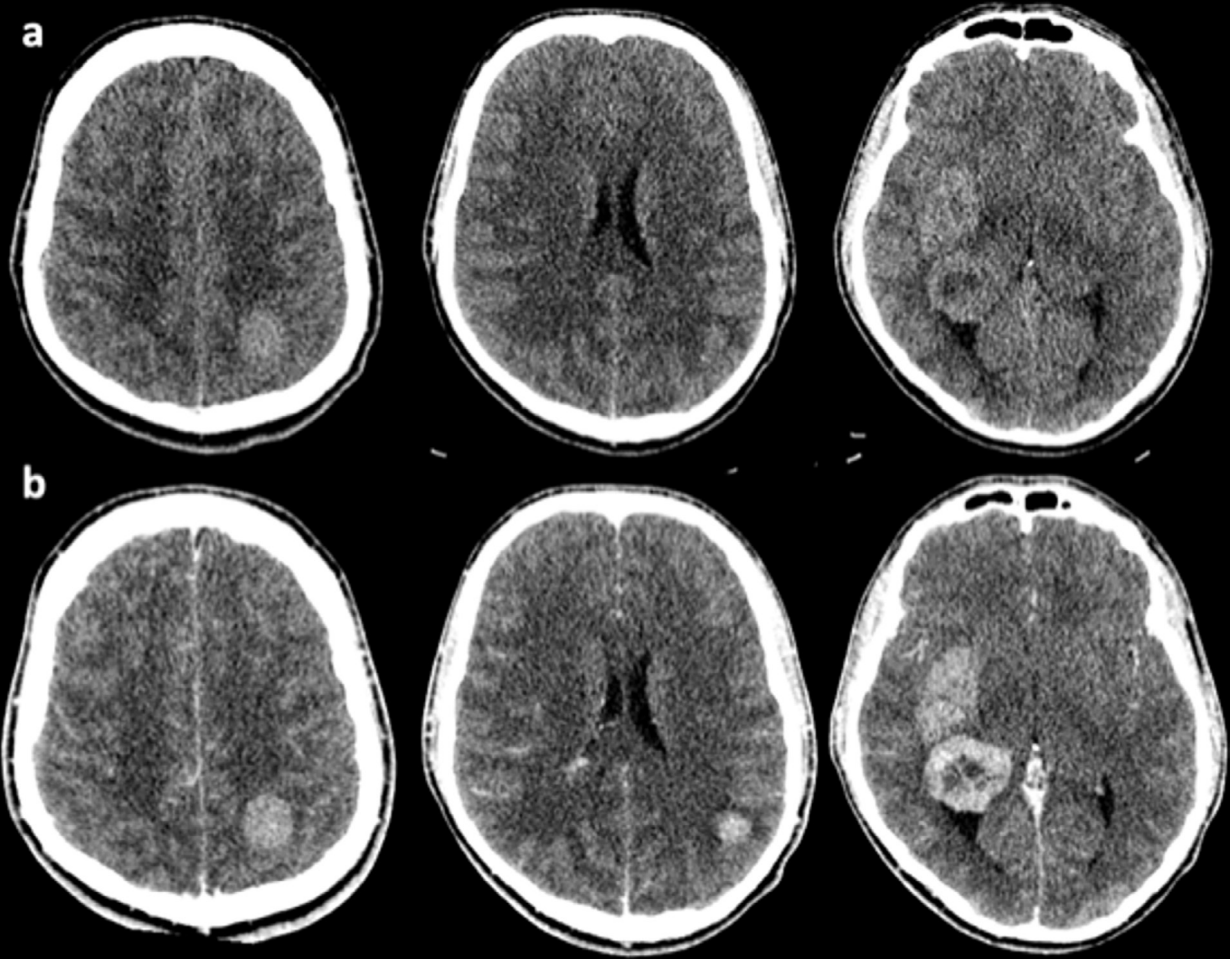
Pre- (a) and post-contrast (b) CT imaging performed on admission demonstrating bilateral cerebral tumours.
